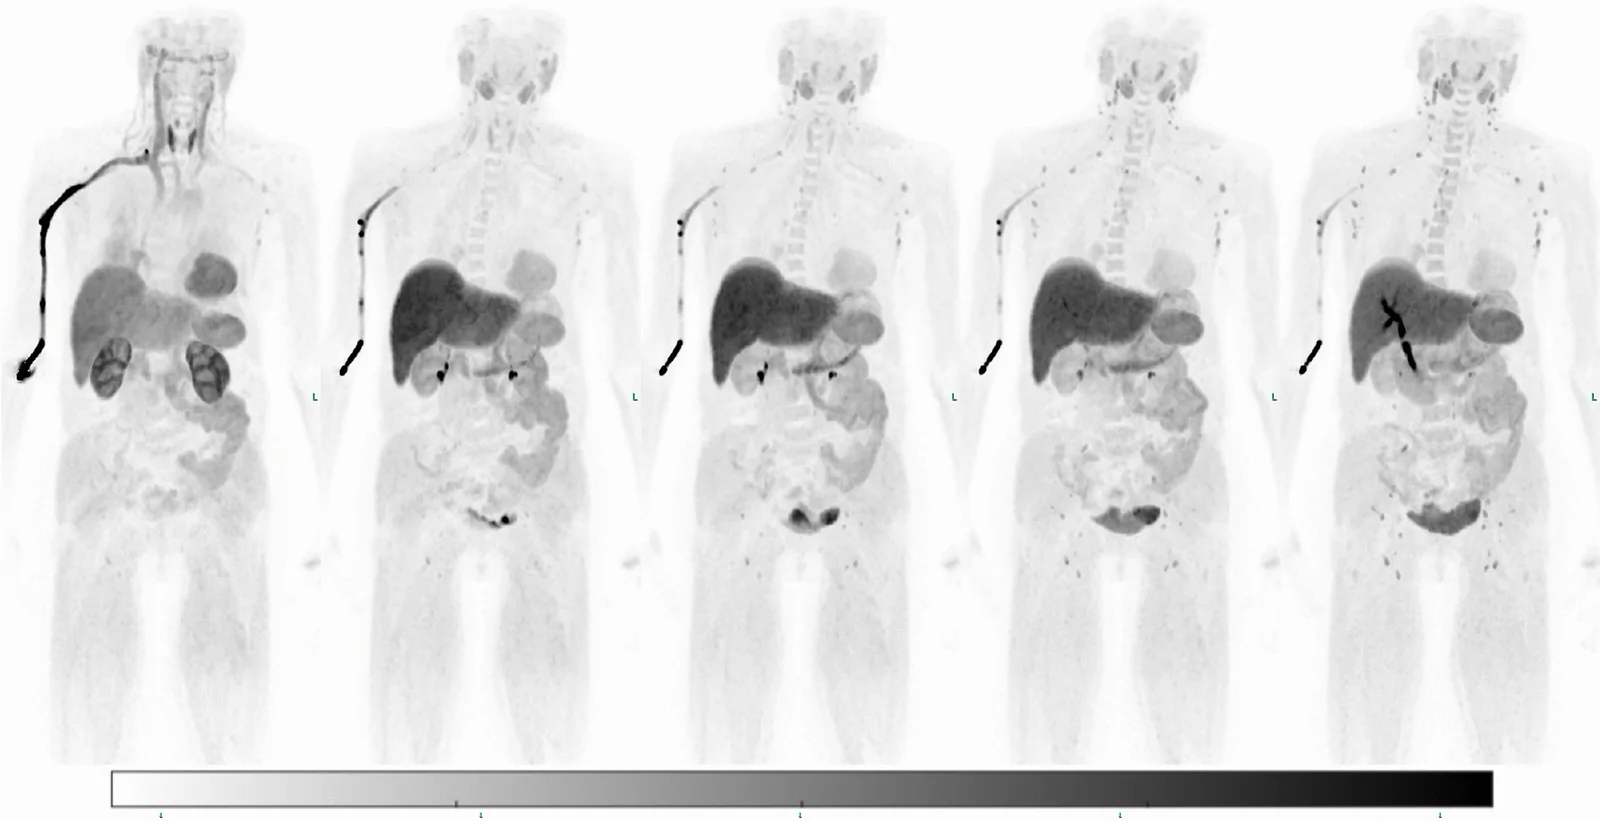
MIP images of a representative participant with ovarian cancer imaged with a ^11^C-PARP1-selective radiopharmaceutical [^11^C]AZ14193391. Acquired PET images with a GE Discovery MI PET/CT. Start of acquisition of the five sequential PET acquisitions were (from left) approximately 0, 10, 20, 30, and 45 min post injection of [^11^C]AZ14193391. All images have equal window settings (SUV scale range 0 – 17.5). Visual evaluation of PET images shows high activity concentration in the gastrointestinal tract, liver, kidneys, red marrow, pancreas, salivary glands, and spleen
